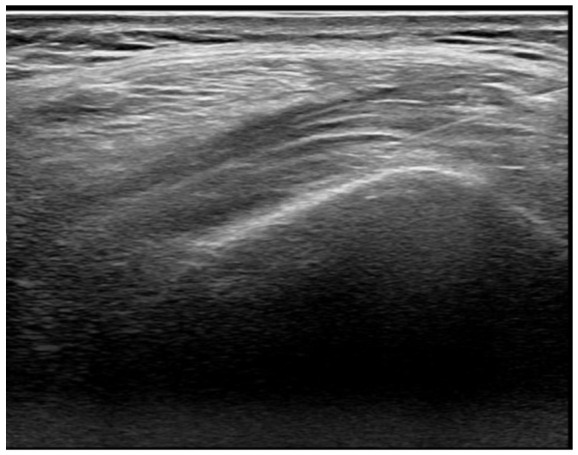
Ultrasound-guided intra-articular knee injection, with the needle visualized throughout its course using an out-of-plane technique, advancing into the subquadricipital recess.
